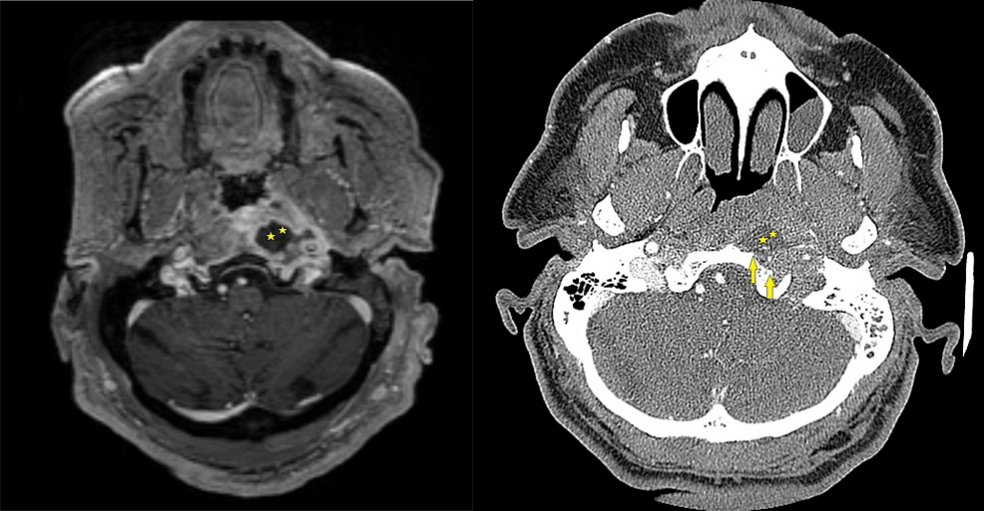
Axial MRI (left) and CT scan (right): anterior clival cortical bone loss (yellow arrows) and preclival rim-enhancing lesion (asterisks).
